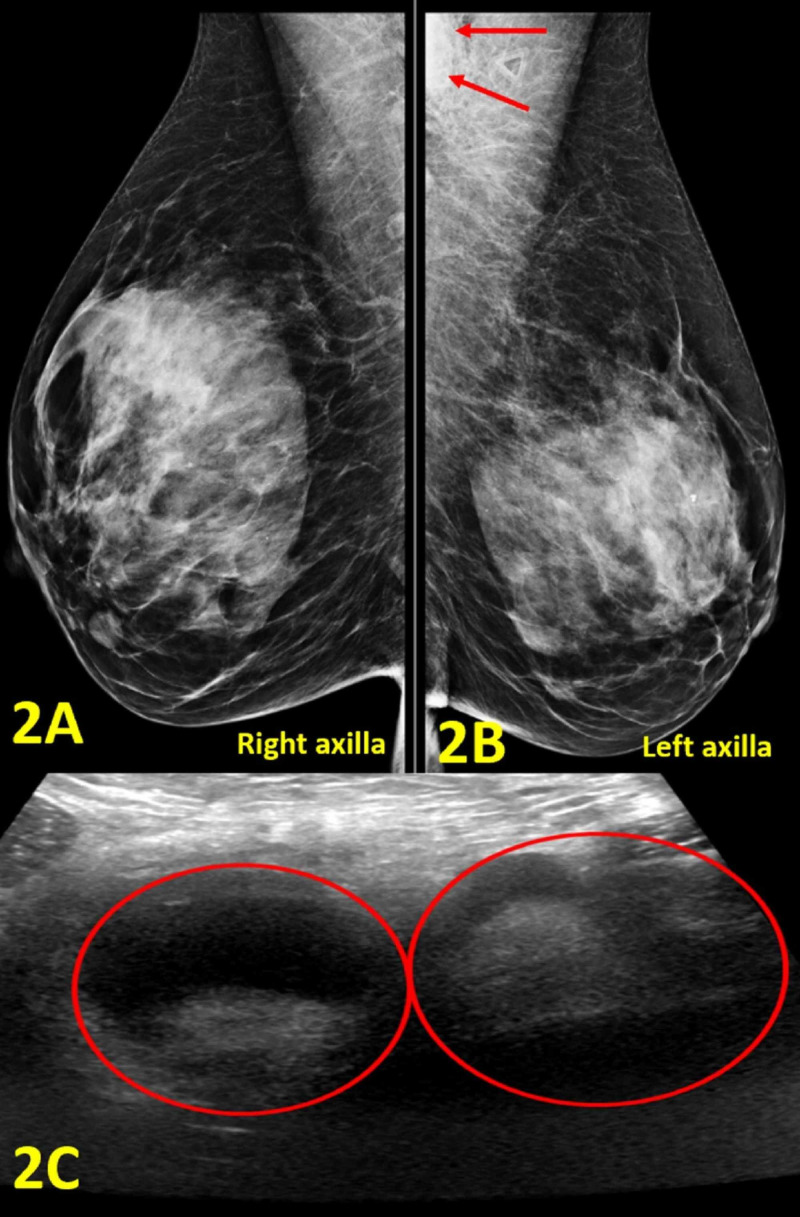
Imaging of Bilateral Breasts and Axillae (A) Right axilla diagnostic mammogram demonstrates no abnormal findings. (B) Left axilla diagnostic mammogram shows an enlarged lymph node in the left axilla (outlined by red arrows). (C) Left axilla diagnostic ultrasound shows two adjacent enlarged axillary lymph nodes with cortical thickening measuring 31 mm and 27 mm in diameter, respectively (red circles).
